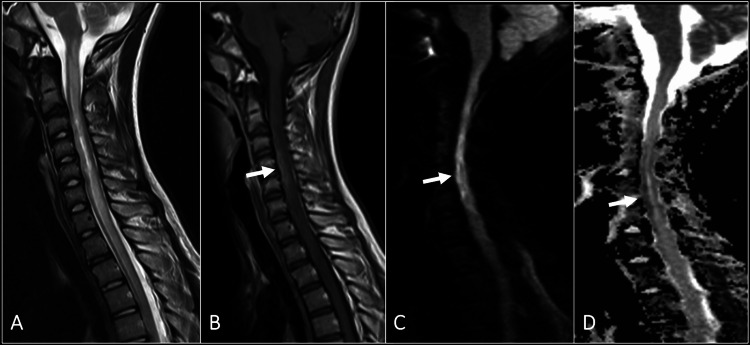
The follow-up MRI after two weeks from the onset: (A) Sagittal T2 image shows interval worsening of the signal abnormality and the swelling of the spinal cord. (B) There is an abnormal enhancement in the anterior cord, which is localized at the same area of abnormal signal intensity in the initial imaging (arrow). (C) Sagittal diffusion-weighted image and (D) ADC map show diffusion restriction in the anterior cord from C3 to C5 (arrows).
